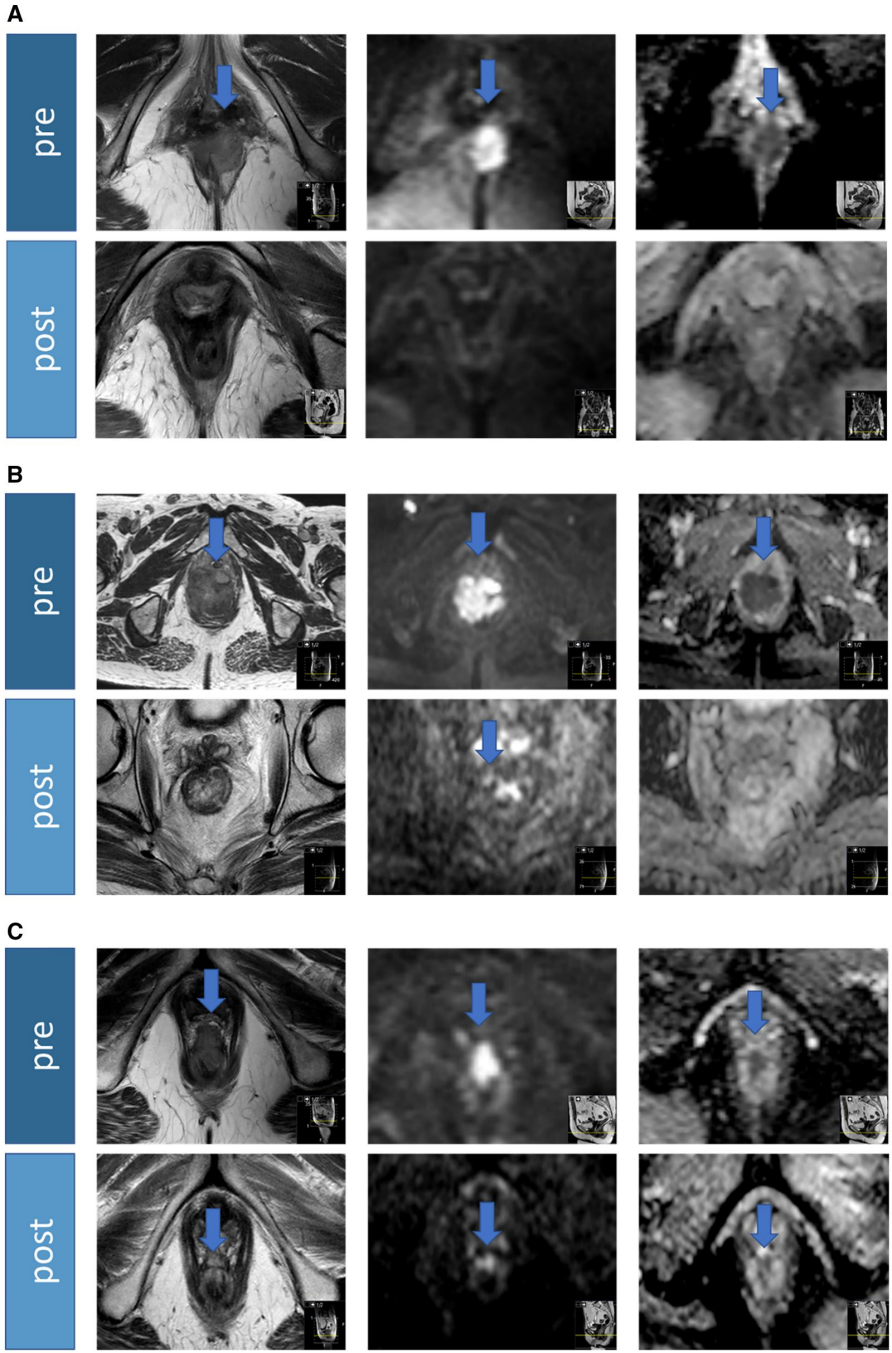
Imaging examples of tumour regression grade based on T2+diffusion weighted MRI (comrTRG) score 1-3. Images show transversal T2WI (left), DWI b800 (mid), and ADC (right) before and after treatment with CRT. (A) comrTRG1: complete response/no tumour, (B) comrTRG2: near-complete response/indeterminate tumour, and (C) comrTRG3: moderate respons/minor definite tumour in predominant fibrosis. Blue arrows mark the tumor. Abbreviations: comrTRG = combined MR tumour regression grade; ADC = apparent diffusion coefficient; DWI = diffusion-weighted imaging; T2WI = T2-weighted imaging.
